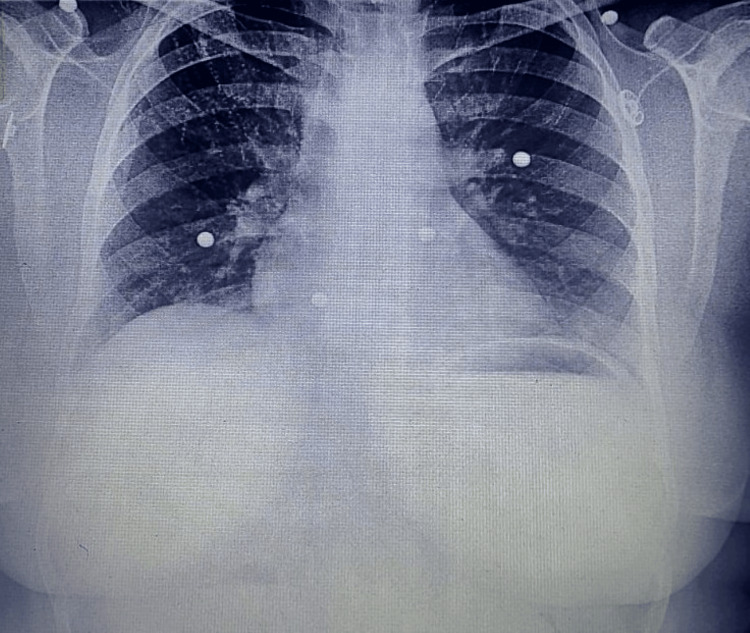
Posteroanterior view chest X-ray demonstrating no acute cardiopulmonary findings
